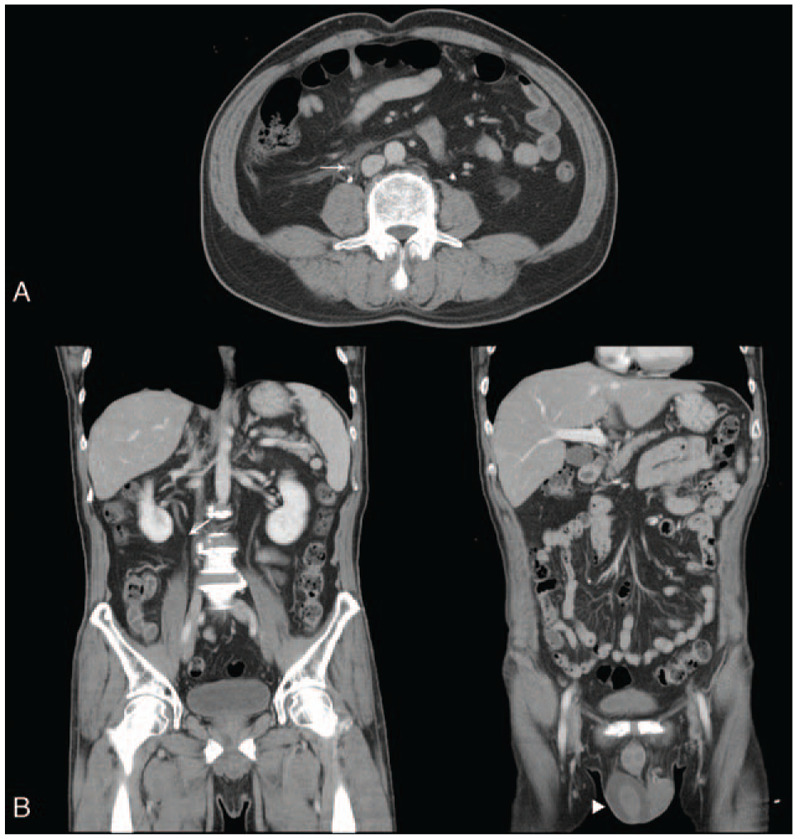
Abdominal computed tomography scan. (A) An axial view showed retroperitoneal fat stranding and minimal fluid at the level below pancreas. Note right testicular vein (arrow) was closely surrounded by adjacent inflammatory tissue. (B) Coronal section demonstrated that the proximal part of right testicular vein (arrow) was obscured by retroperitoneal fluid and edematous tissue. It further led to scrotal hydrocele (arrowhead). There was no massive fluid extending from abdomen to the pelvis. Bilateral psoas muscles were visible.
